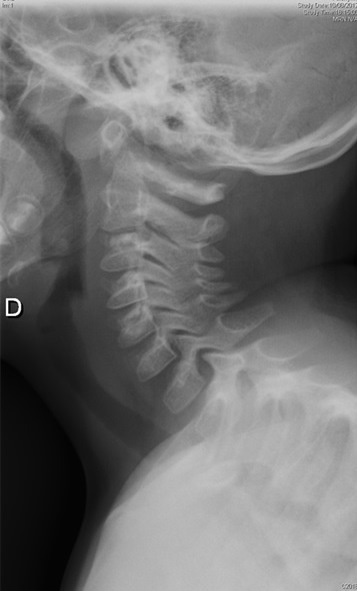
Radiographie du rachis cervical incidence de profil ou l’on voit au niveau des vertèbres C2C3 des calcifications discales étagées des disques intervertébraux chez le patient 1
